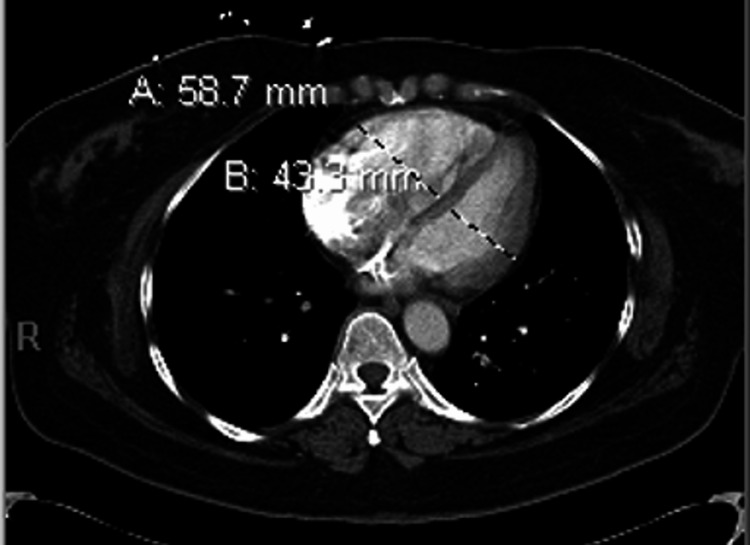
A computed tomography angiogram of the chest (axial images) shows an asymmetric enlargement of the right ventricle compared to the left and mild flattening of the interventricular septum, suggesting right heart strain.
